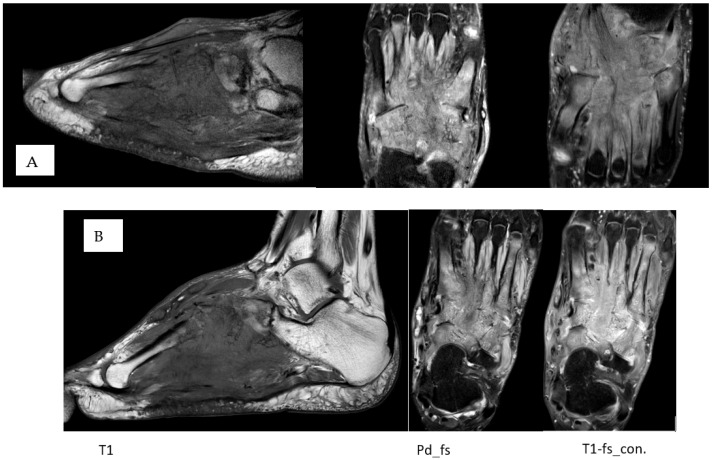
MRI before the bone biopsy (A) and repeated after treatment (B) showed that the findings remained stable.
